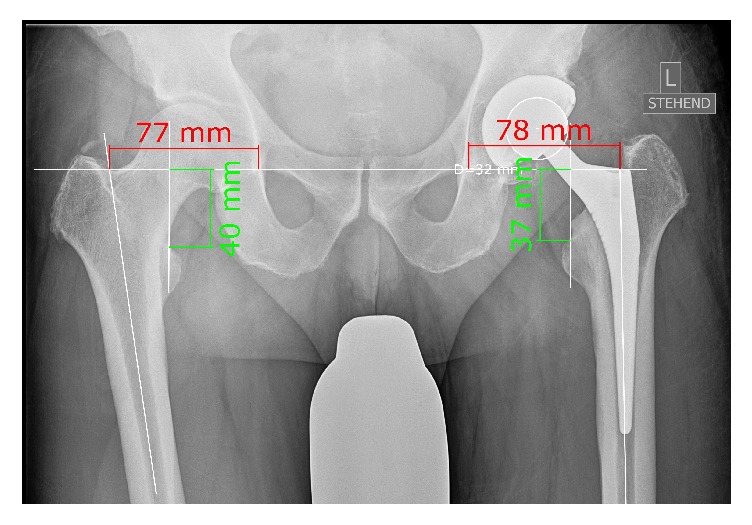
Postoperative assessment of residual leg length and offset differences in relation to the contralateral side on postoperative radiographs.
